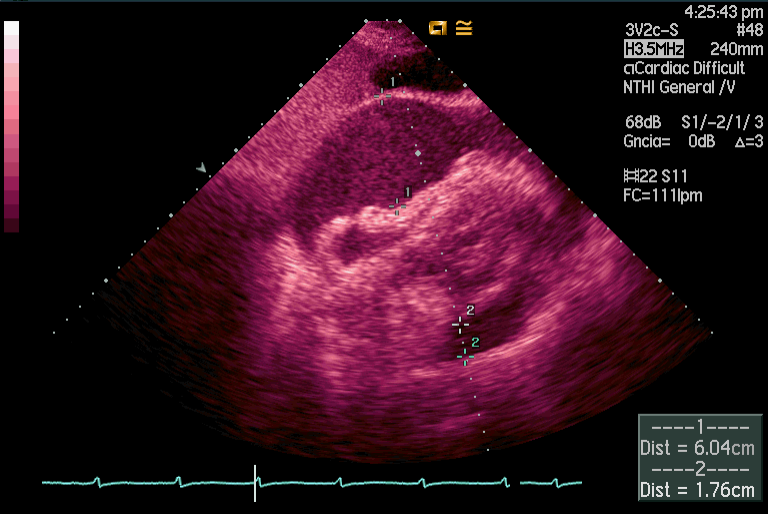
Severe pericardial effusion in subcostal transthoracic view.
